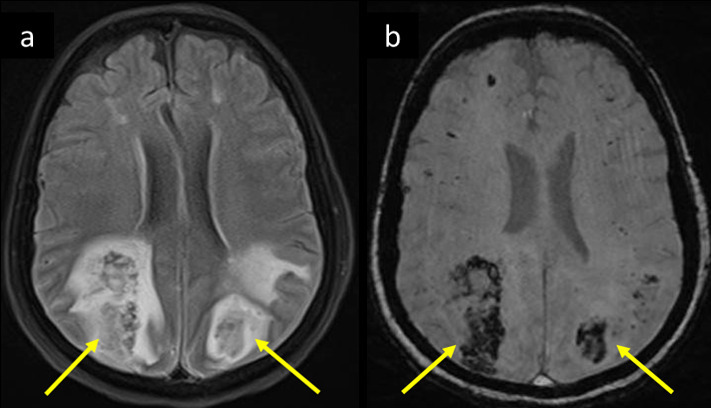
Same patient as in Figure 6 showed progressive deterioration of GCS. Her follow-up MRI 3 days later, axial (a) *T*_2_-FLAIR shows bilateral parietooccipital white matter vasogenic edema with bright signal intensity, and hemorrhagic changes showing central areas of low signal intensity consistent with hemorrhagic PRES. Corresponding blooming of hemorrhages are seen on (b) magnitude image of SWI (arrows in a and b). GCS, Glasgow coma scale;FLAIR, fluid attenuated inversion recovery; PRES, posterior reversibleencephalopathy syndrome; SWI, susceptibility-weighted imaging.
